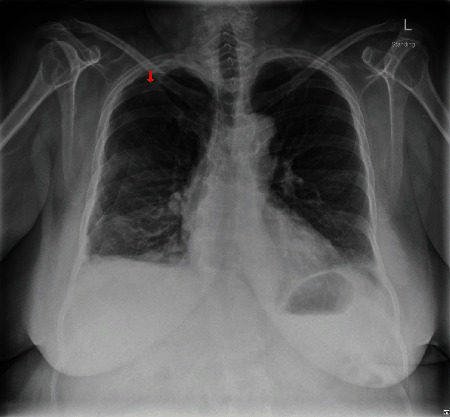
Chest X-ray showing moderate right-sided apicolateral pneumothorax (depicted by red arrow).
